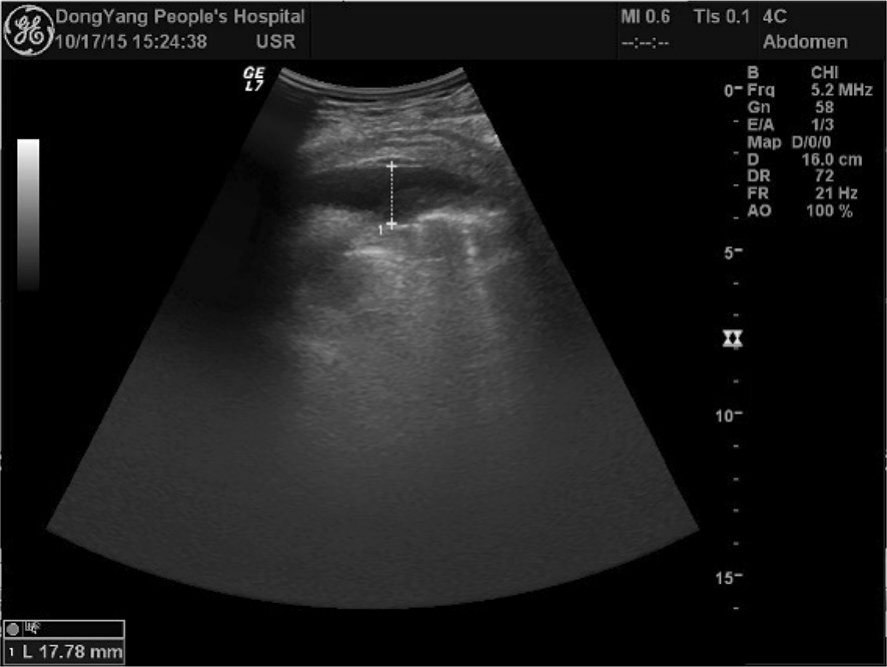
Ultrasound on October 17 indicated that bilateral bulk pleural effusion was almost completely disappeared (2 months later)
